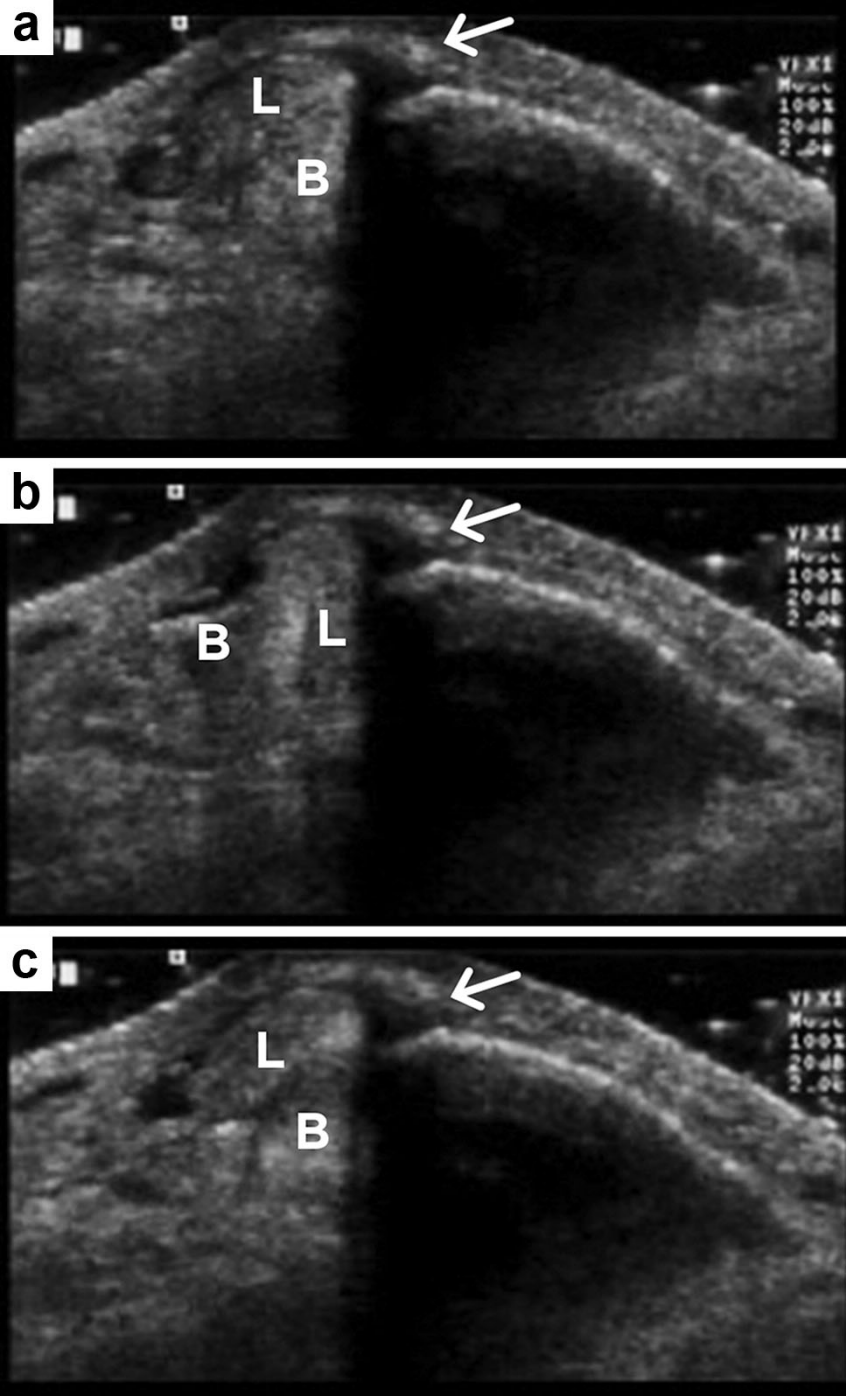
Retromalleolar intrasheath peroneal tendon subluxation in a young downhill skier occult at MRI and demonstrated at dynamic ultrasound examination. a Transverse ultrasound scan at the level of the lateral malleolus with the ankle at rest shows the peroneus longus (L) and peroneus brevis, (B) tendons in anatomical position. The *arrow points* at a mildly thickened superior retinaculum. b During active ankle eversion, the tendons abruptly interchange position within the retromalleolar groove. Note the increased laxity of the superior retinaculum (*arrow*). c As the ankle is brought into neutral position, the tendons suddenly switched back to their anatomical position. (*arrow* = superior retinaculum)
